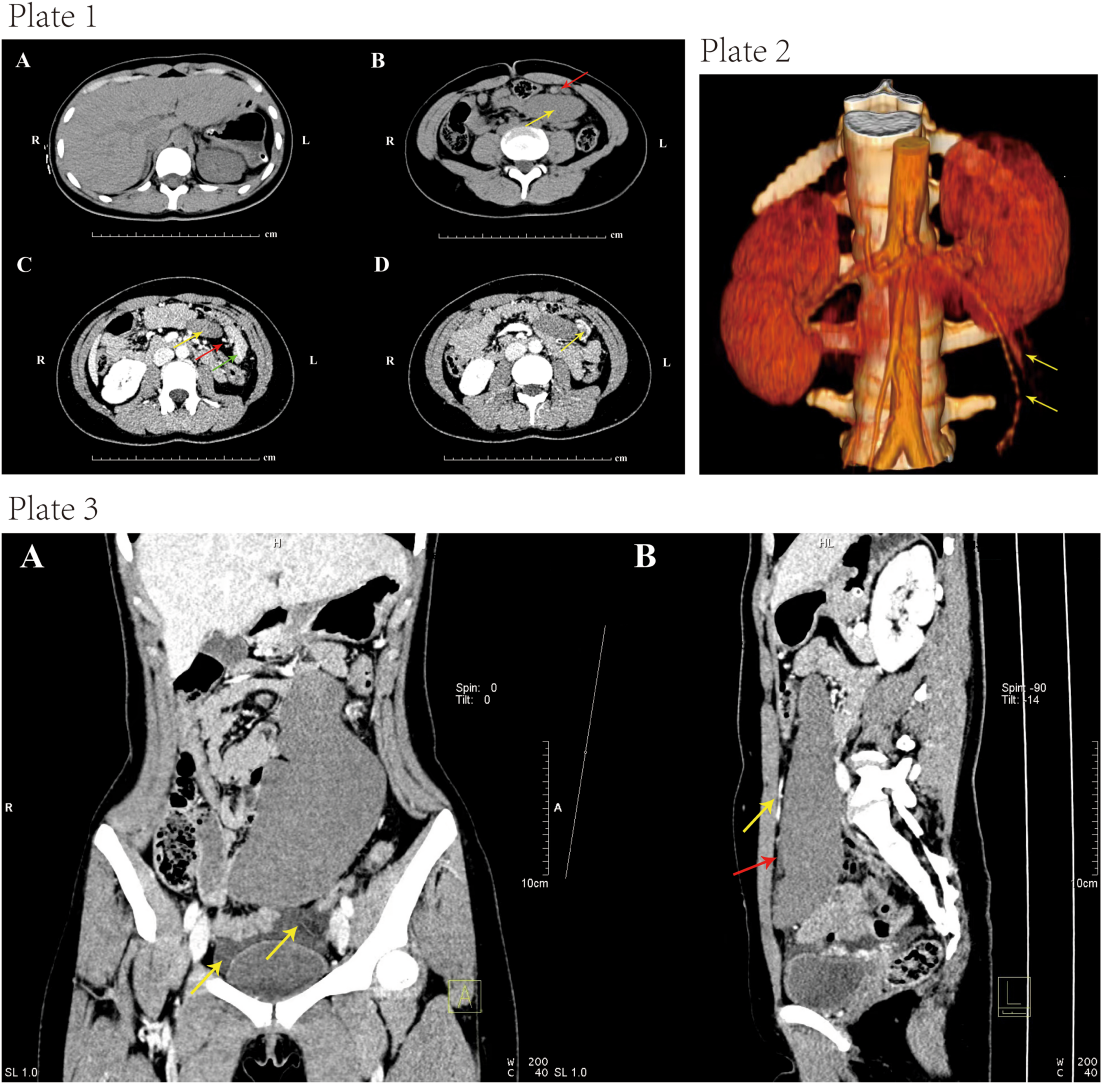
Plate 1, contrast-enhanced computed tomography (CECT) images. (A) Absence of the spleen in the left upper abdomen after plain CT scan. (B) CT scan indicated an elliptic soft tissue density shadow (yellow arrow) in the left upper quadrant. The hyperdense shadows in splenic veins suggested splenic vein thrombosis (red arrow). (C) The axial section showed a “whirl sign” (red arrow) of the splenic pedicle vessels counterclockwise, together with pancreatic tail torsion (green arrow). The white arrow indicated the absence of obvious enhancement in the spleen, showing a possibility of splenic infarction. (D) The visualized splenic veins showed dilatation and tortuosity (yellow arrow). Plate 2, computed tomography (CT) image showing elongated splenic pedicle vessels (yellow arrow). Plate 3, coronal and sagittal CT images. (A) Contrast-enhanced CT scan indicated splenomegaly. There was pelvic fluid induced by splenic infarction. (B) There was a filling of contrast agent in the upper segment of the vessels (yellow arrow), while there was no filling in the lower segment (red arrow). This indicated a block of the splenic artery.
